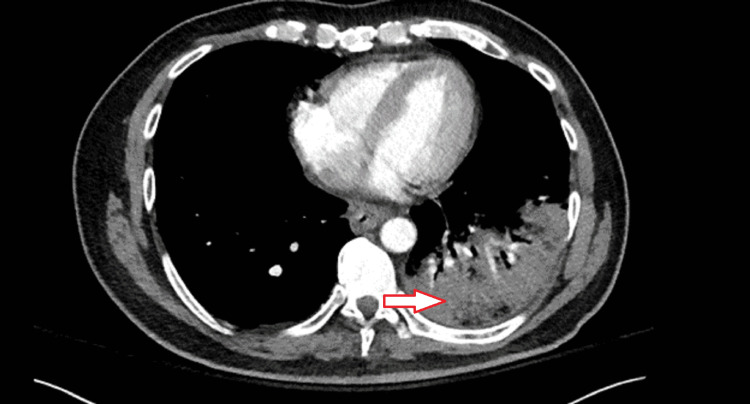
CT angiogram of the chest The red arrow indicates an area of airspace consolidation on the lower lobe of the left lung
